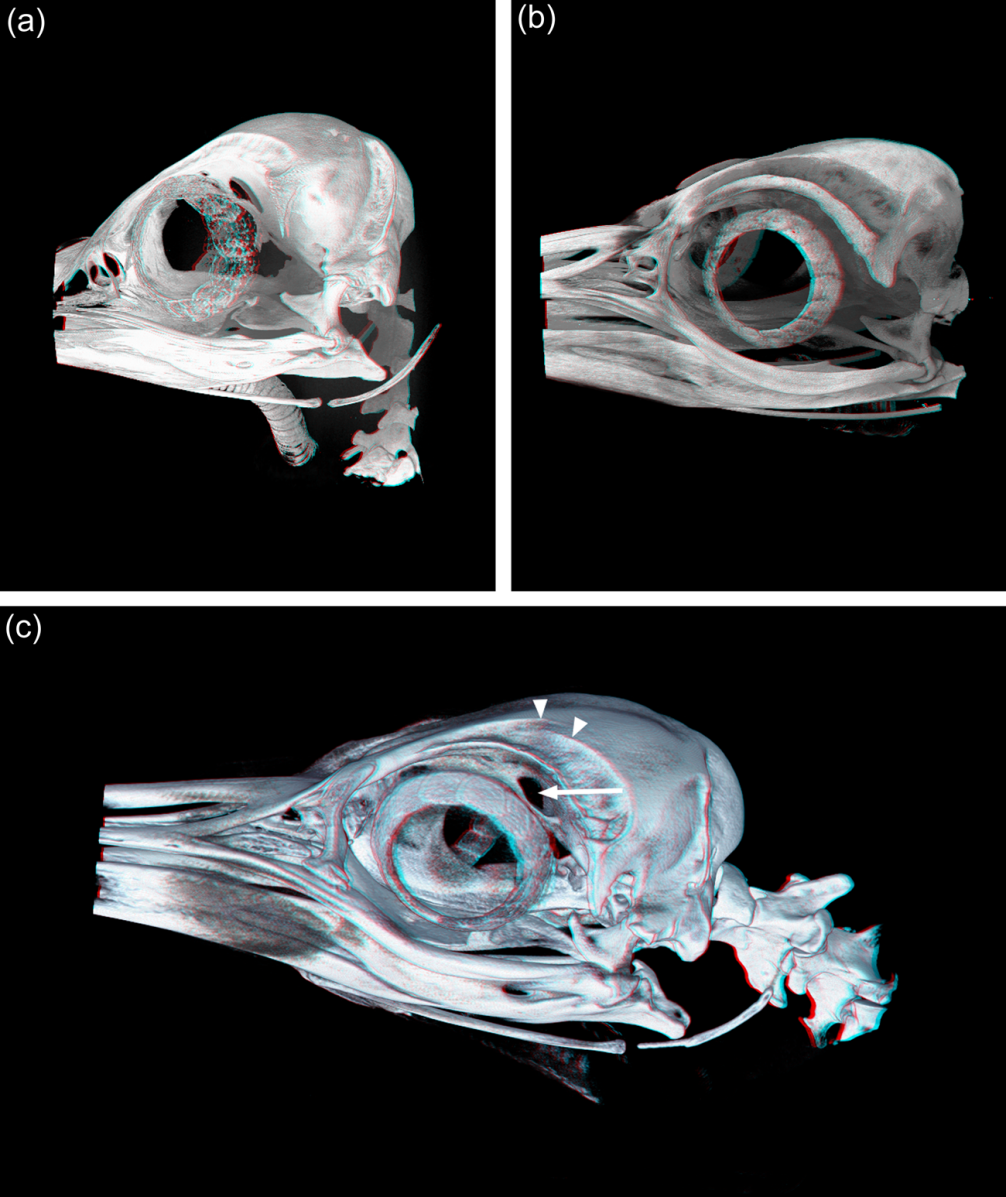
Three‐dimensional CTVox reconstructions of frozen head micro‐CT scans. (a) Little penguin (*Eudyptula minor*) L1. (b) Gentoo penguin (*Pygoscelis papua*) G1. (c) King penguin (*Aptenodytes patagonicus*) K1. (a) and (b) are approximately left lateral views. The view of the king penguin (c) is of the right side of the skull and has been rotated 180° around the *y* axis for ease of comparison with the other figures. It has also been rotated 10° around the *x* axis to display a foramen in Paries caudalis orbitae (arrow). These images may be viewed stereoscopically using red‐green glasses. The detail visible in K1 is markedly lower than that visible in L1 or G1 due to the lower resolution scanner required to image the larger head. There is also an artificial discontinuity present at the level of the mid orbit of K1, due to transient vibration during the imaging process in a factory environment (the arrowheads mark the medial border of Fossa glandulae nasalis, which should be continuous)
